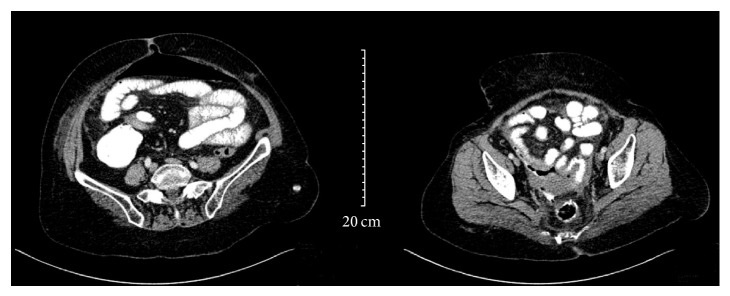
CT of the abdomen, showing extravasation of luminal contrast from the rectosigmoid region into a collection in the pelvis and air bubbles around the anastomosis. There is associated free air in the peritoneum.
